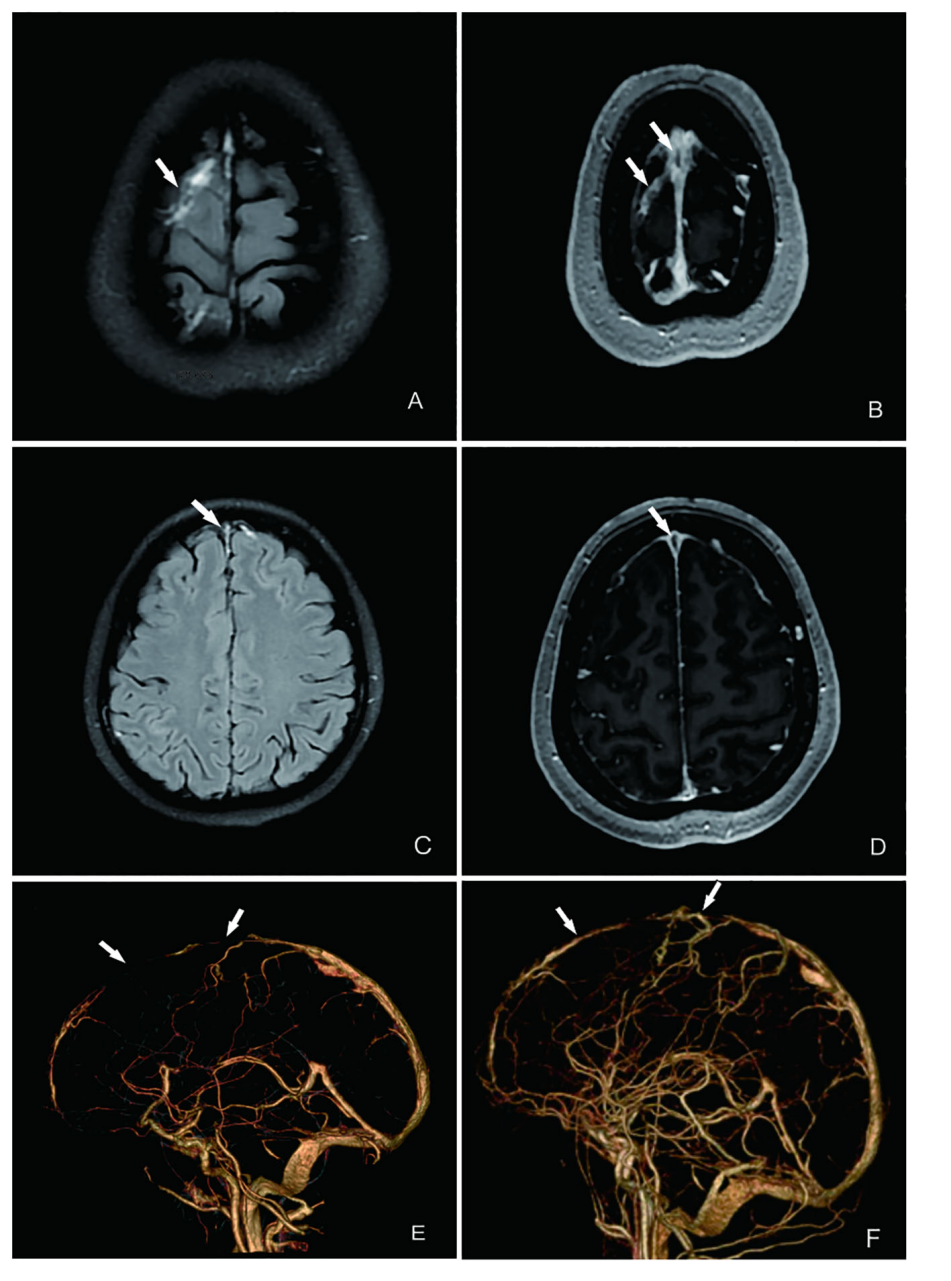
MRI and CTV of the patient’s brain showing multiple intracranial thrombosis in the venous sinus. (A) T2 imaging suggests multiple superficial venous thrombosis (indicated by the arrow). (B) T1-enhanced imaging showing the same results as those shown in (A). (C) T2 flare imaging indicating superior sagittal sinus thrombosis (indicated by the arrow). (D) T2-enhanced imaging showing the same results as those shown in (C). (E) CTV showing a filling defect in the superior sagittal sinus on CTV, indicating thrombosis formation. (F) After treatment of CTV, partial resolution of the superior sagittal sinus thrombus and improvement in thrombus severity are seen, compared with before treatment.
